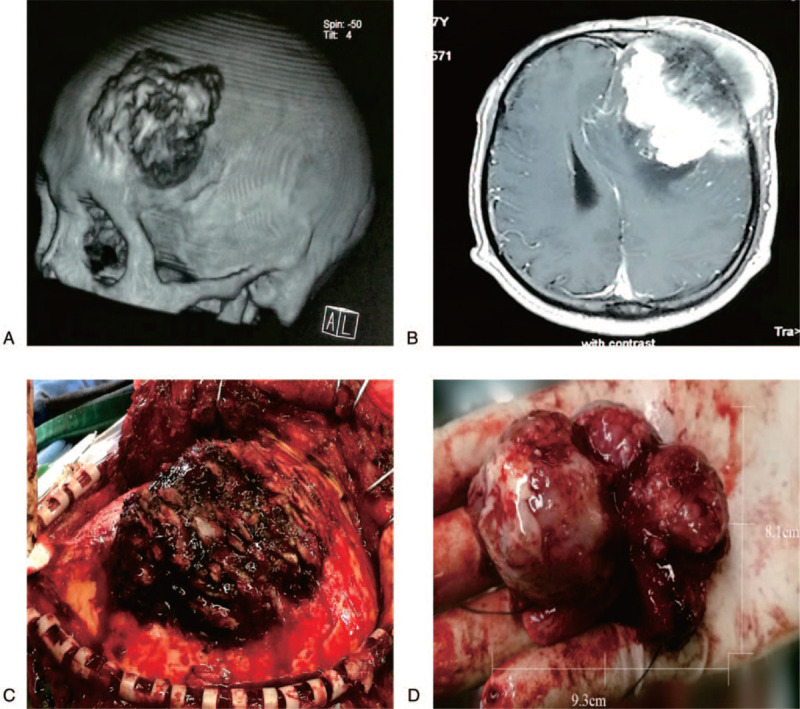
Preoperative skull CT/MRI scan and intraoperative photographs during resection and debulking. (A: Irregular bone destruction of the left frontal bone, the range is about 5.7 cm × 6.3 cm. B: Irregular masses are seen in the left frontal cranial and subscalp, the size is about 4.9 cm × 5.4 cm, it is uneven and obviously strengthened, and the center line structure is skewed to the right. C: The outer plate of skull, raised bone (4.8 cm × 5.2 cm) surface of the skull's outer plate is uneven, showing cancellous bone. D: The tumor isolated from the operation is hard in texture, about 5.7 cm × 6.3 cm in size.).
